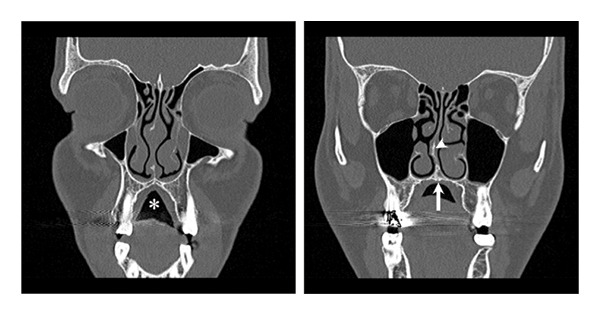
Noncontrasted CT scan of the paranasal sinuses, coronal view, showing a constricted high‐arched palate (star), wide maxillary crest which is a potential area for palate injury (arrow), deviated nasal septum (arrowhead), and hypertrophied inferior turbinates.
